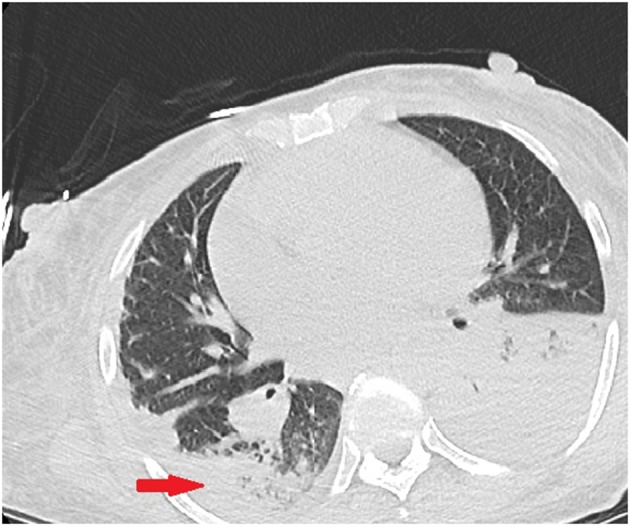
Initial CT chest without contrast demonstrating left lower lobe infiltrate and pleural effusion. Right lower lobe showing patchy reticulonodular interstitial lung disease.
